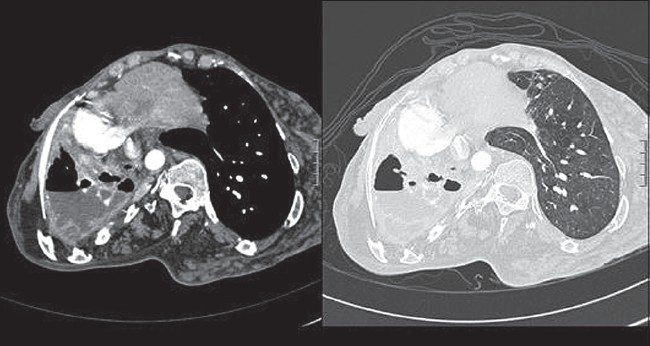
Computed tomography of the chest revealed marked collapse of the right lung, ipsilateral shift of mediastinum with bronchiectatic changes within the collapsed lung. It also showed thick and calcified rind, loculated pleural effusion with air-fluid level and right sided rib thickening. The left lung parenchyma was normal
